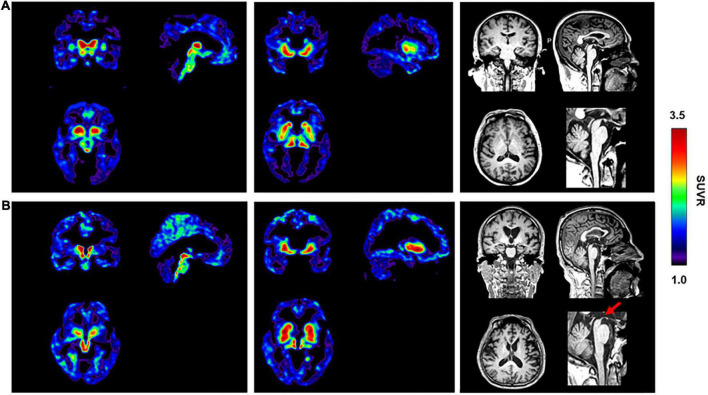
[^18^F]-S16 retention in an elderly healthy control [male, MMSE = 30, (A)] and a patient with progressive supranuclear palsy [male, MMSE = 18, PSP, (B)]. Transverse, sagittal and coronal sections of [^18^F]-S16 PET SUVR images were observed with considerable radioactive retention in the midbrain and basal ganglia. The typical “hummingbird sign” was detected on the sagittal T1 weighted image of the patient (red arrow).
